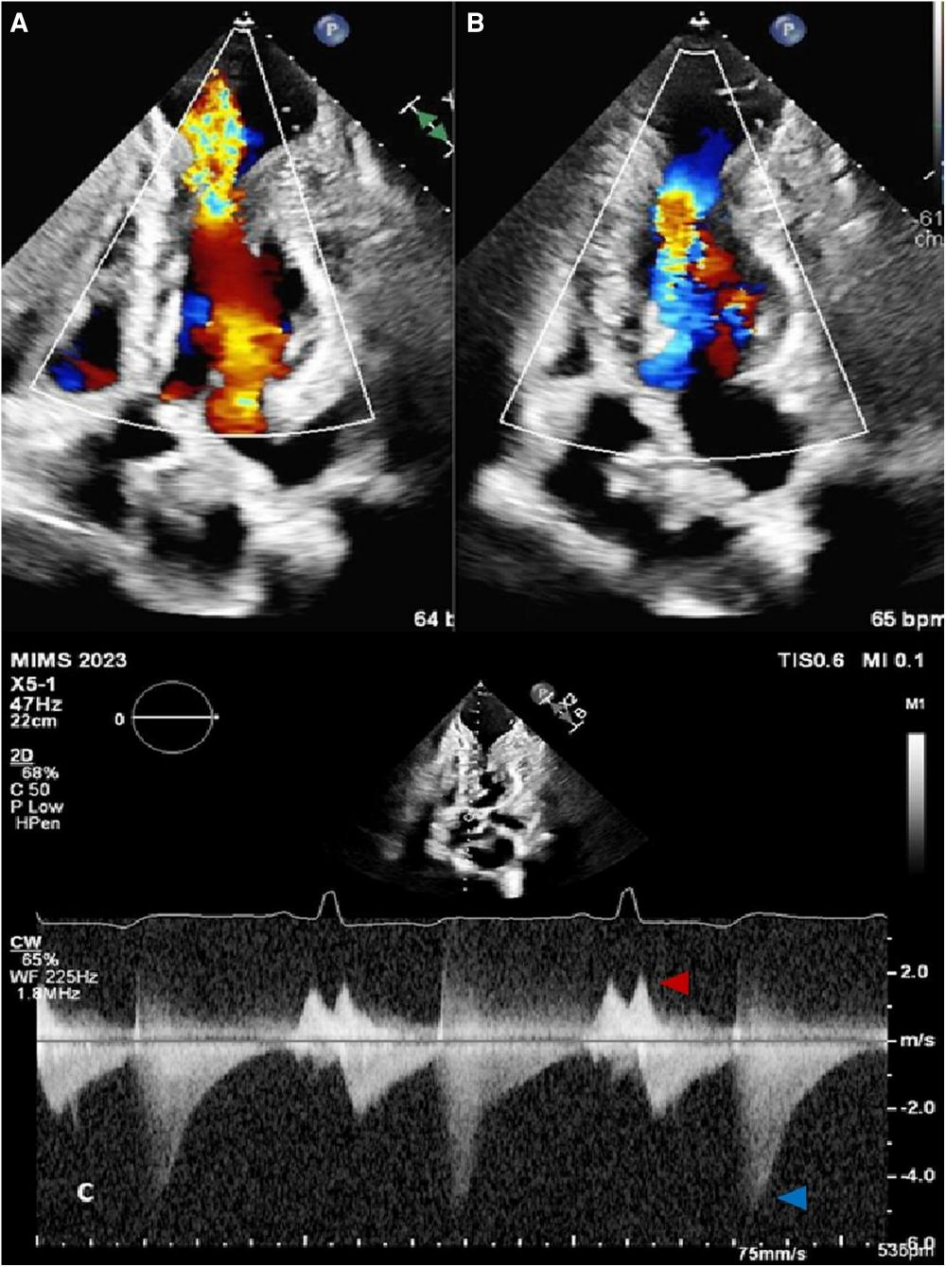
(A*) Colour Doppler showing paradoxical systolic flow into the apical aneurysm, and *(B)* paradoxical diastolic flow from apex to base. *(C*) Continuous wave Doppler revealing paradoxical bifid systolic flow (red arrow), and prominent reverse diastolic flow of 5 m/s towards the base (blue arrow).
